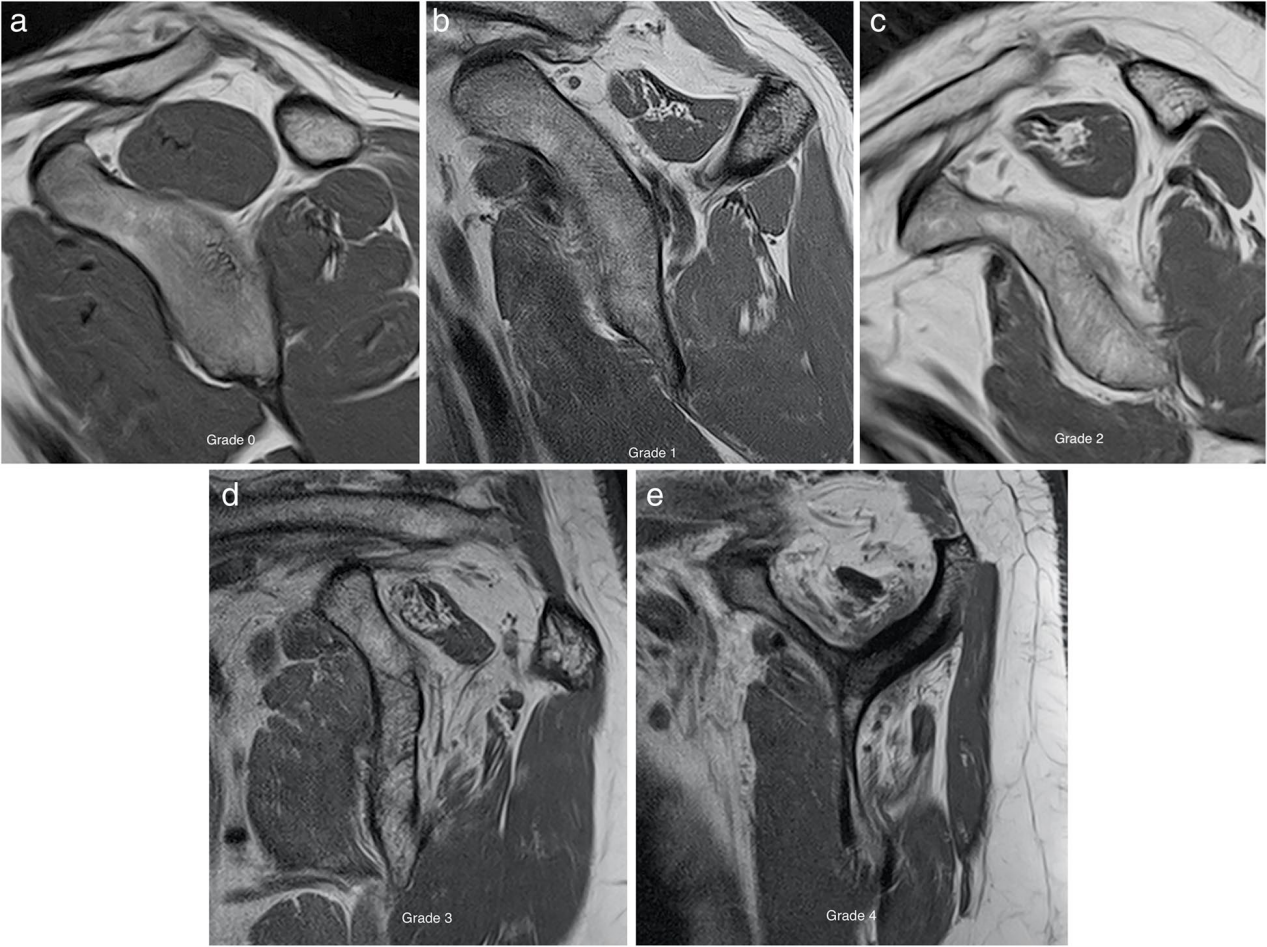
Fatty infiltration. a–e Oblique sagittal T1-weighted MRI showing the different grades of fatty atrophy following Goutallier modify classification for SS and IS tendons. Grade 0 = normal muscle, grade 1 = some fatty streaks, grade 2 = less than 50% fatty muscle atrophy (more muscle than fat), grade 3 = 50% fatty muscle atrophy, (equal muscle and fat), and grade 4 = more than 50% muscle atrophy (more fat than muscle)
